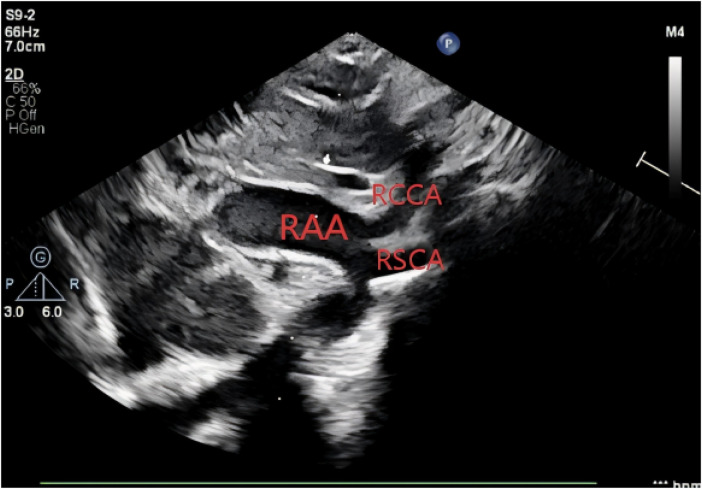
Echocardiographic findings at 1 month of age showing two branches arising from the right aortic arch (RAA, right aortic arch; RCCA, right common carotid artery; RSCA, right subclavian artery).
